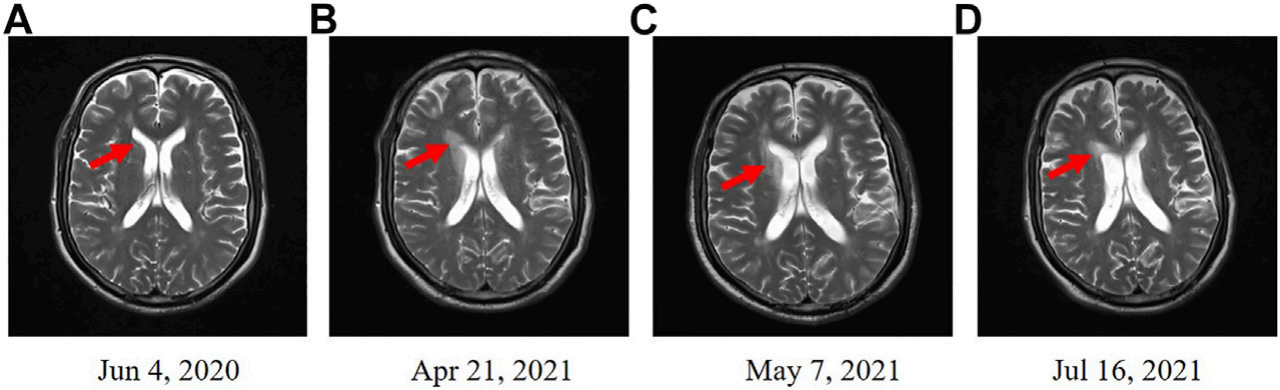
Enhanced MRI scans in head. (A) Enhanced MRI before the treatment of durvalumab. (B) Enhanced MRI revealed the diffuse abnormal signal after a 11-cycle monotherapy maintenance therapy with durvalumab. (C) Enhanced MRI revealed an improved condition after 21 days treatment of methylprednisolone. (D) Enhanced MRI indicated that the diffuse abnormal signal was disappeared after 3 months.
